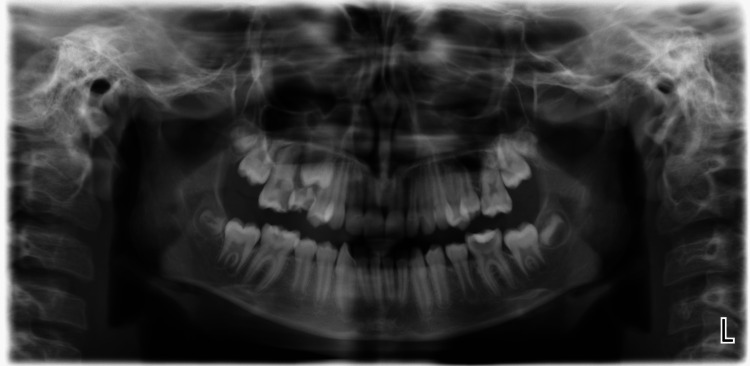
Orthopantomogram from two years ago
